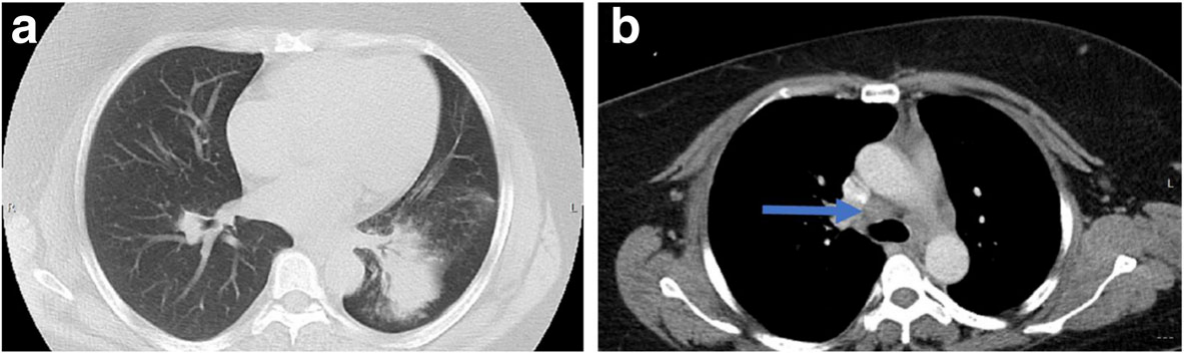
Chest computed tomography reveals a large left lower lobe lung mass. On admission, the patient was found to have (a) a 6.7 × 5.4 × 9.9-cm left lower lobe mass and (b) hilar lymphadenopathy (*arrow*)
